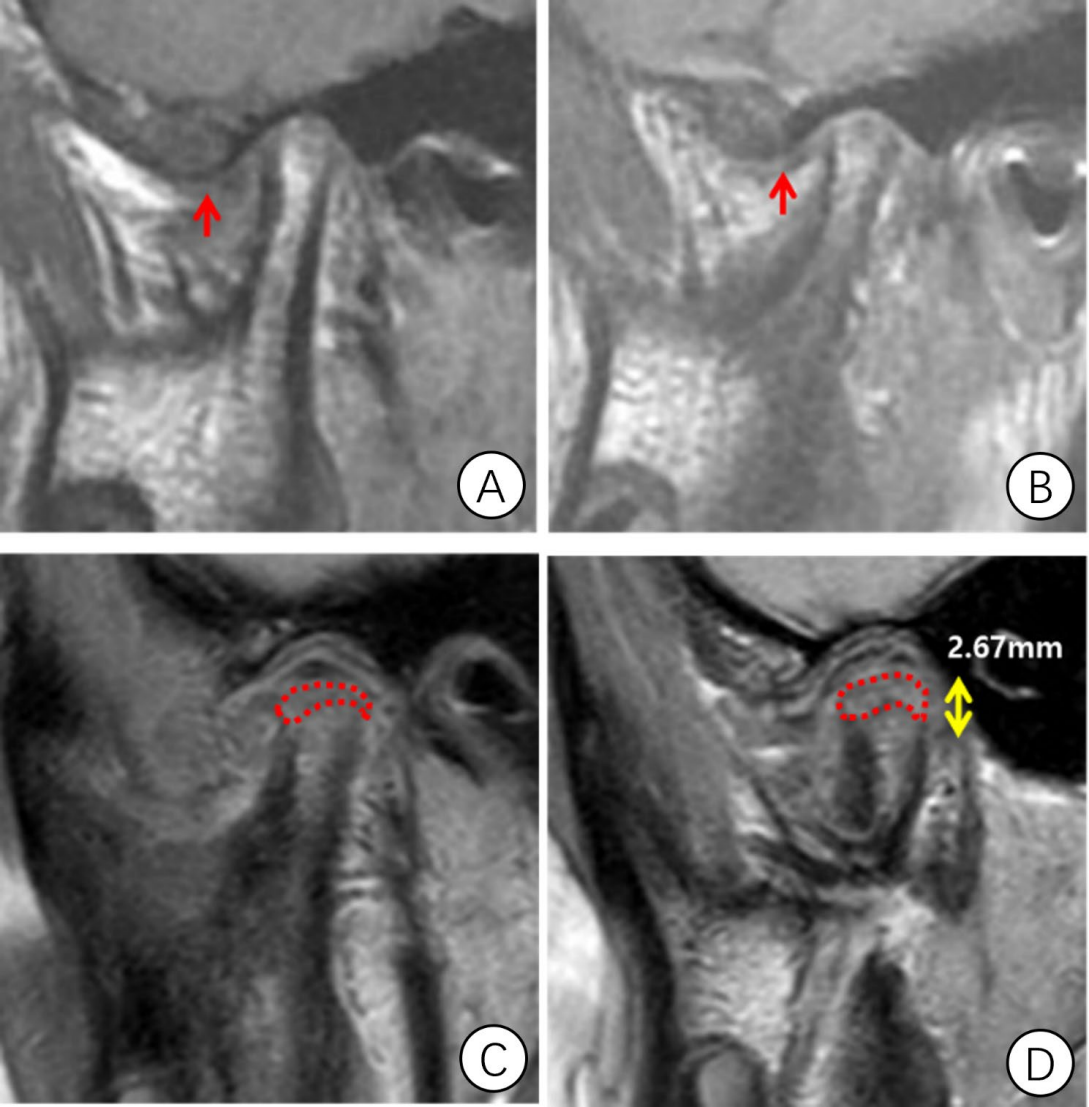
MRI of patient in Fig. 5. A, B, anteriorly displaced disc (red arrows), and discontinuous bone cortex of right & left condyles before operation; C, D, cap-shaped regenerated bone (red dashed line) after 12 months post operation
